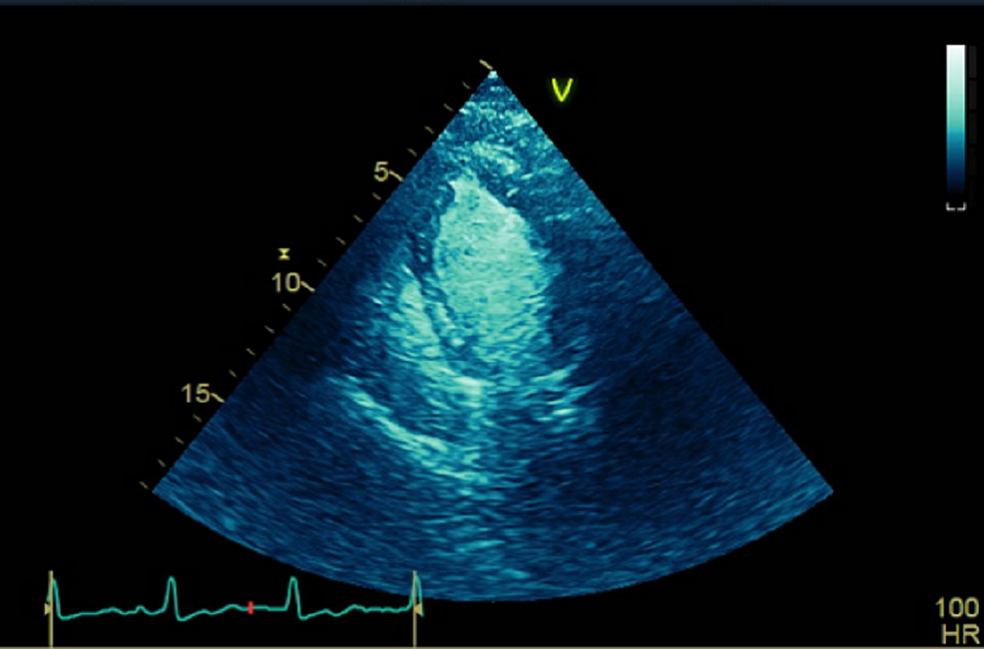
Transthoracic echocardiogram: end systolic, showing contracting left ventricle (LV) apex and mildly dilated base.
